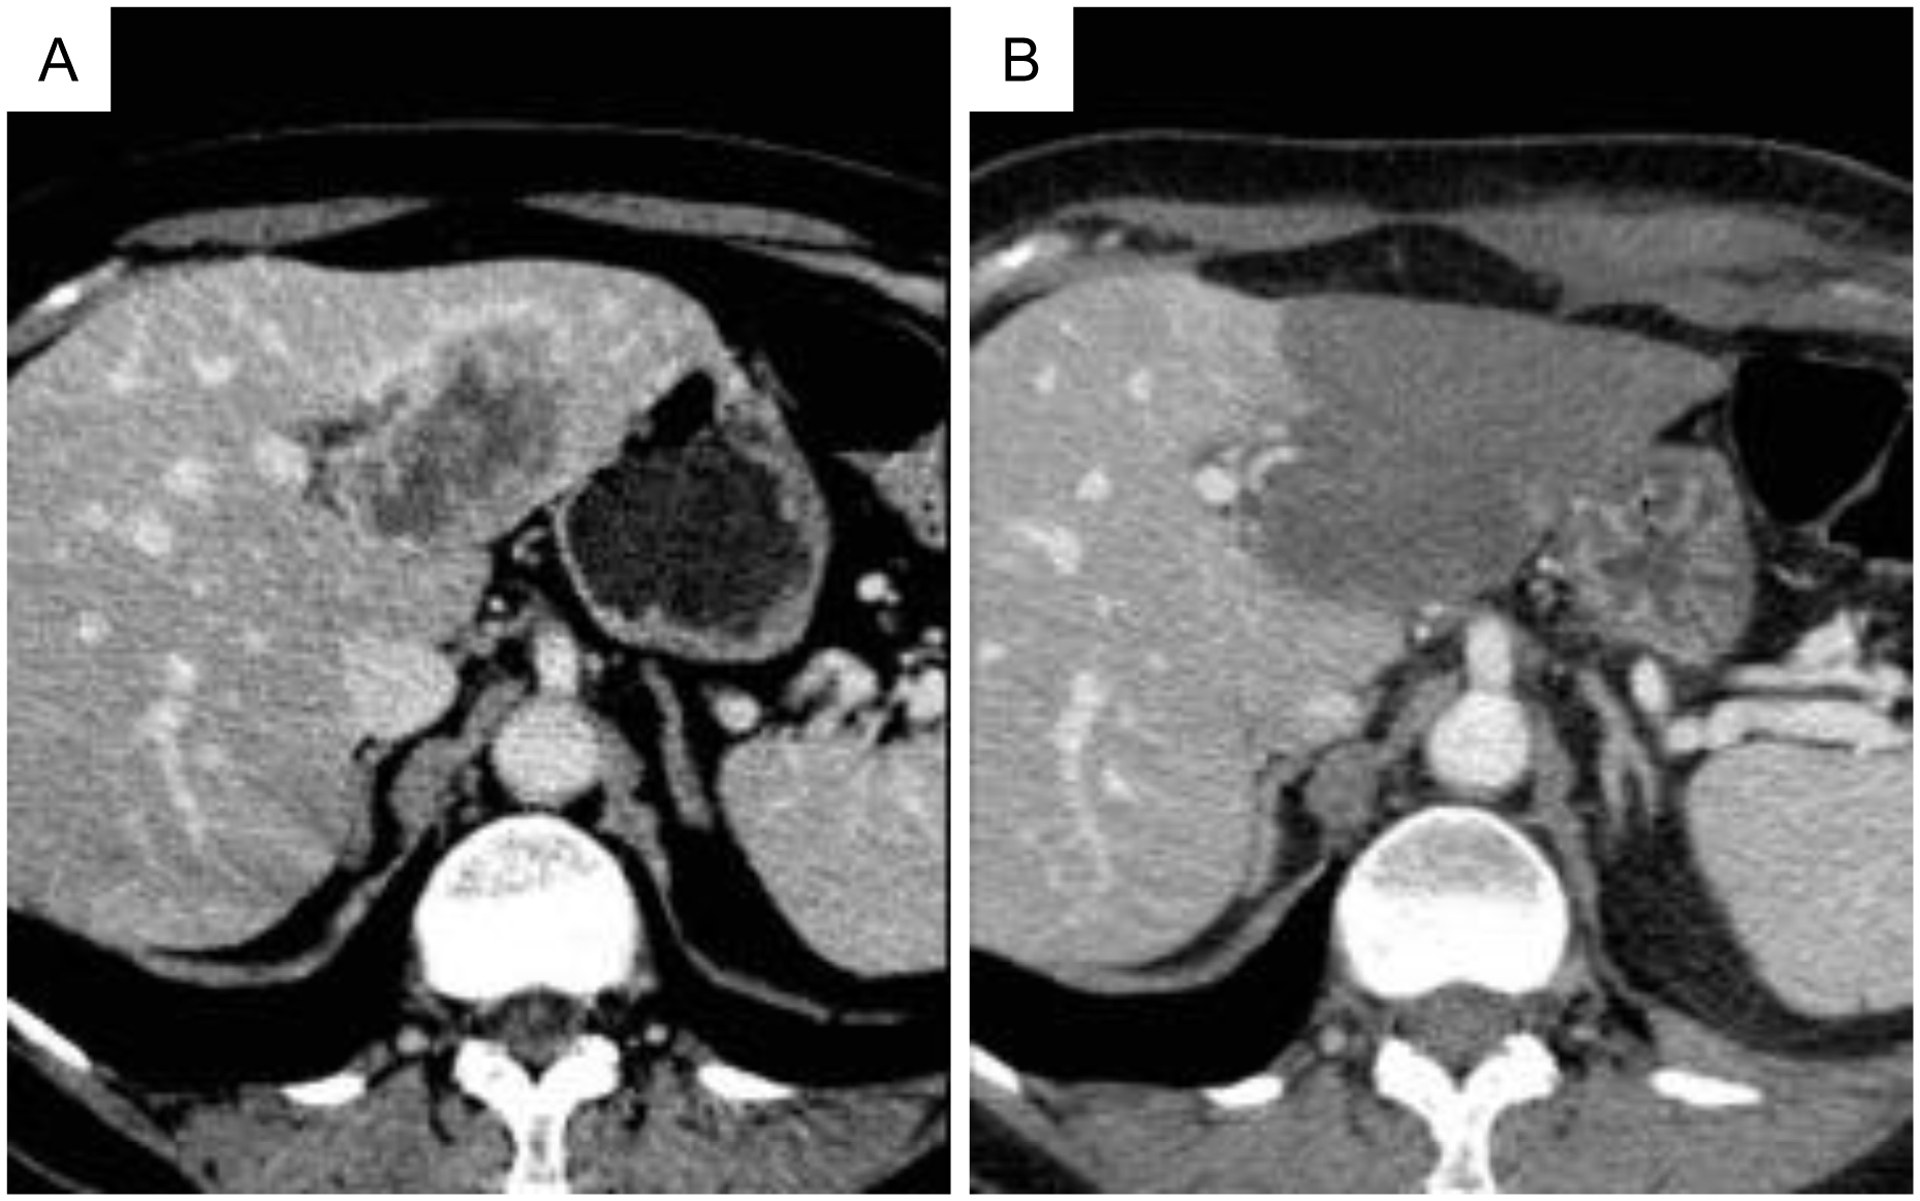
CT imaging pre and postthermal HIFU treatment of the liver. (a) Computed tomography (CT) scan of segment II–III liver metastasis from breast cancer. (b) Contrast-enhanced CT image obtained 24 h following high-intensity focused ultrasound (HIFU) ablation demonstrates a low-attenuating nonenhancing area that has replaced the tumor. No residual tumor enhancement is identified. Figure from [15].
